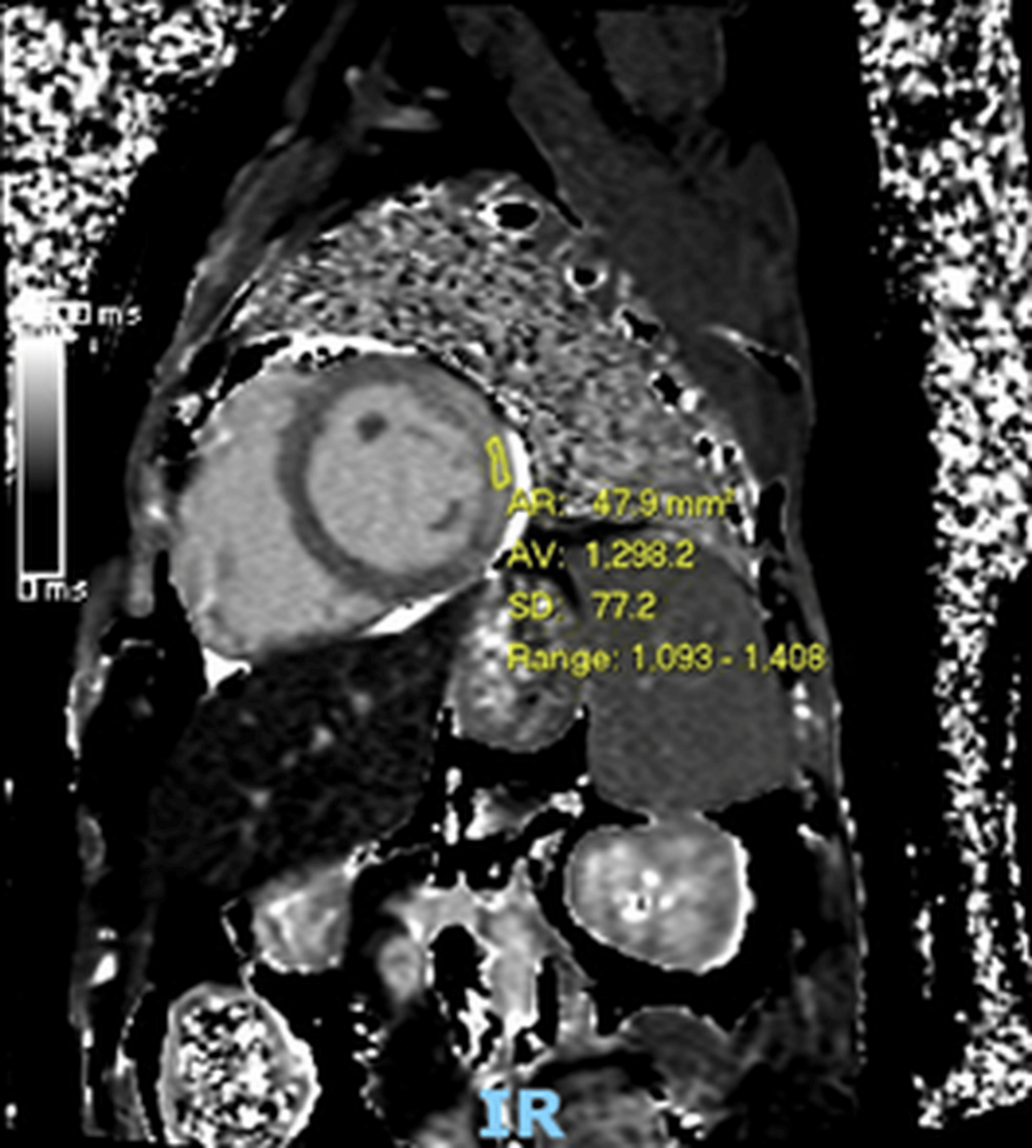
Cardiac MRI demonstrating myocardial oedema and late gadolinium enhancement T2 mapping images show markedly elevated T2 relaxation times, consistent with increased myocardial water content and active inflammation. The short-axis view demonstrates extensive mid-wall and epicardial late gadolinium enhancement involving the lateral wall from base to apex, in keeping with acute myocarditis under the Lake Louise Criteria.
